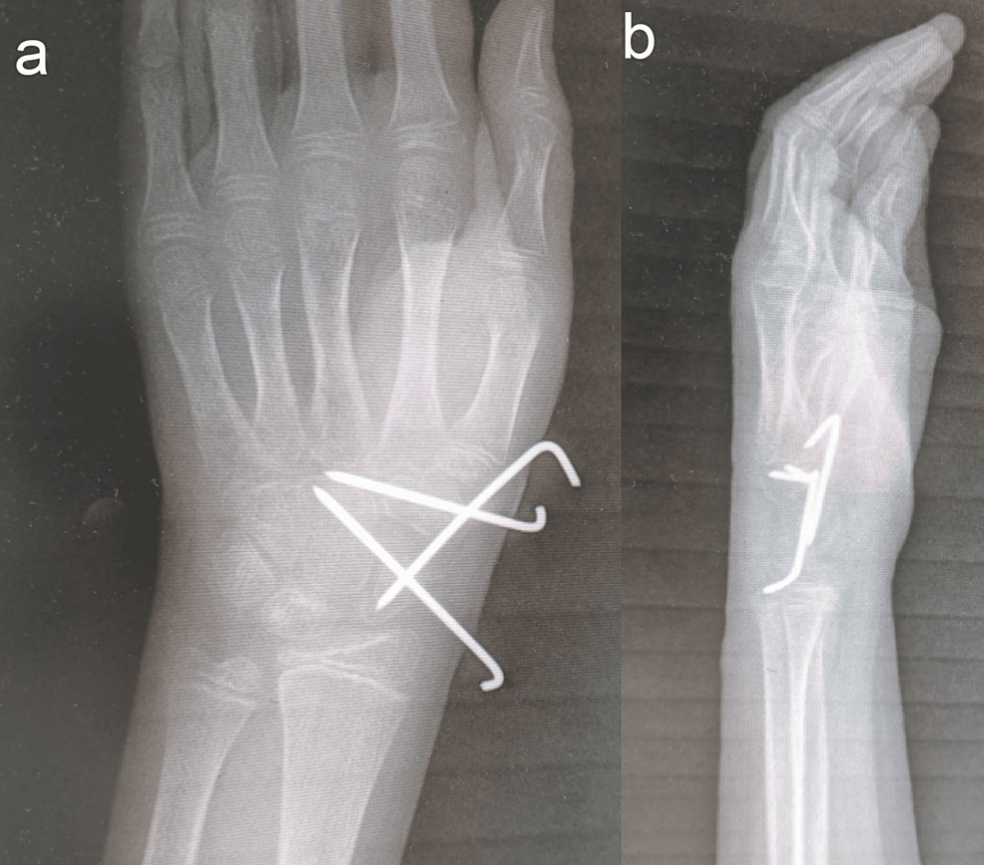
(a) Posterior-anterior and (b) lateral wrist radiographs showed scaphoid-trapezoid-trapezoidal wiring.
